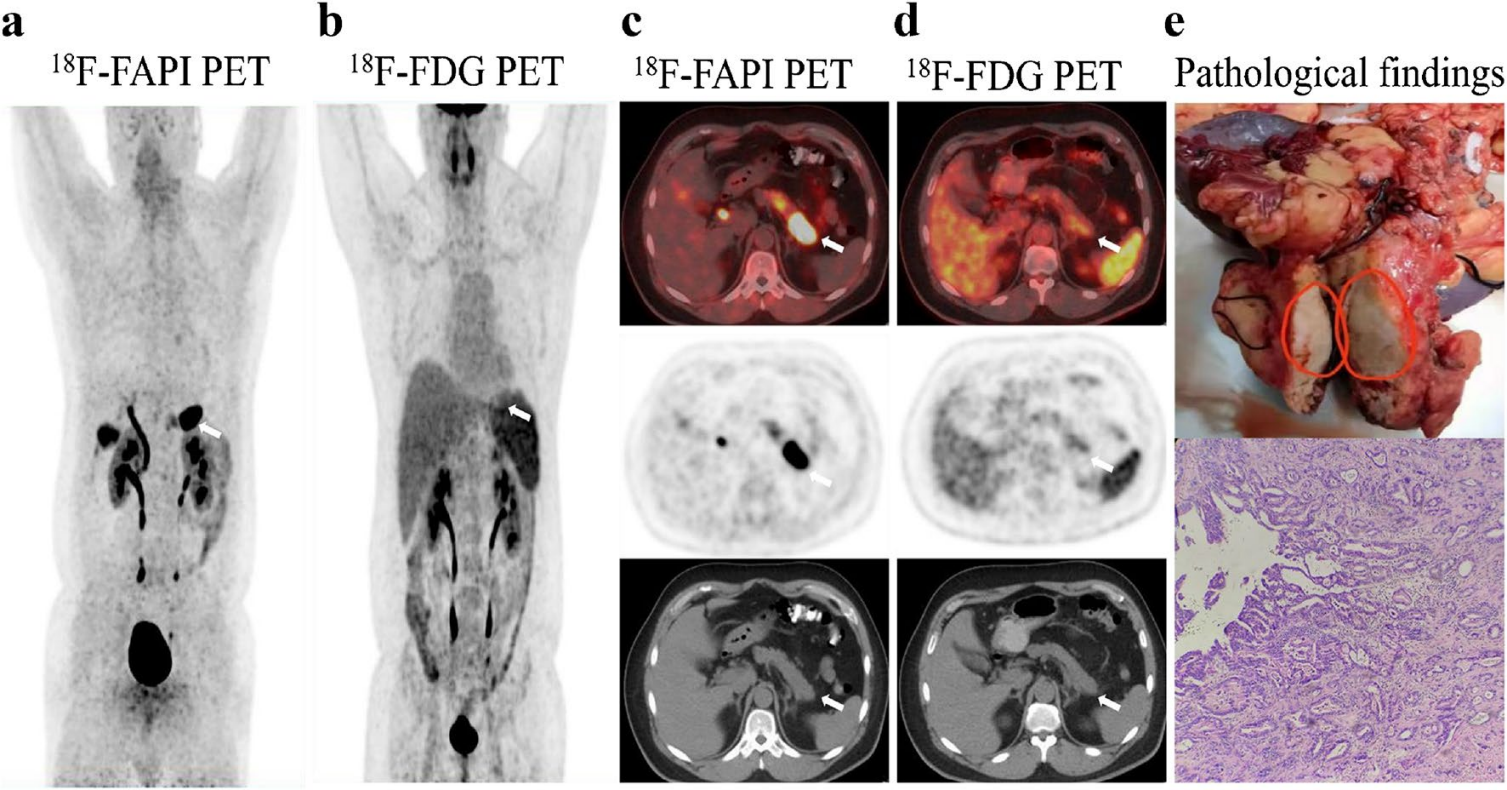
A 43-year-old male underwent PET/CT to evaluate a pancreatic mass previously detected through ultrasound. a, c
